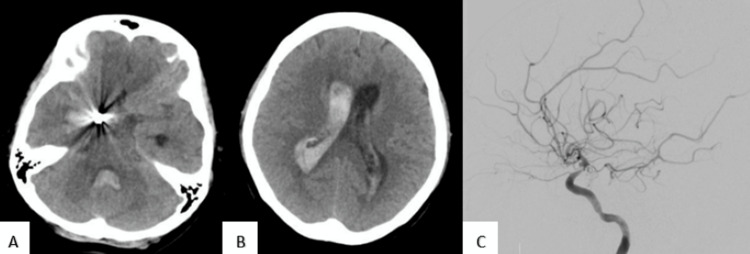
Non-contrast computed tomography brain showed right temporal intracerebral haemorrhage at the site of the coiled aneurysm (A) with intraventricular extension (B); Catheter angiogram showed a blood blister-like aneurysm arising from the dorsal wall of internal carotid artery (C)
